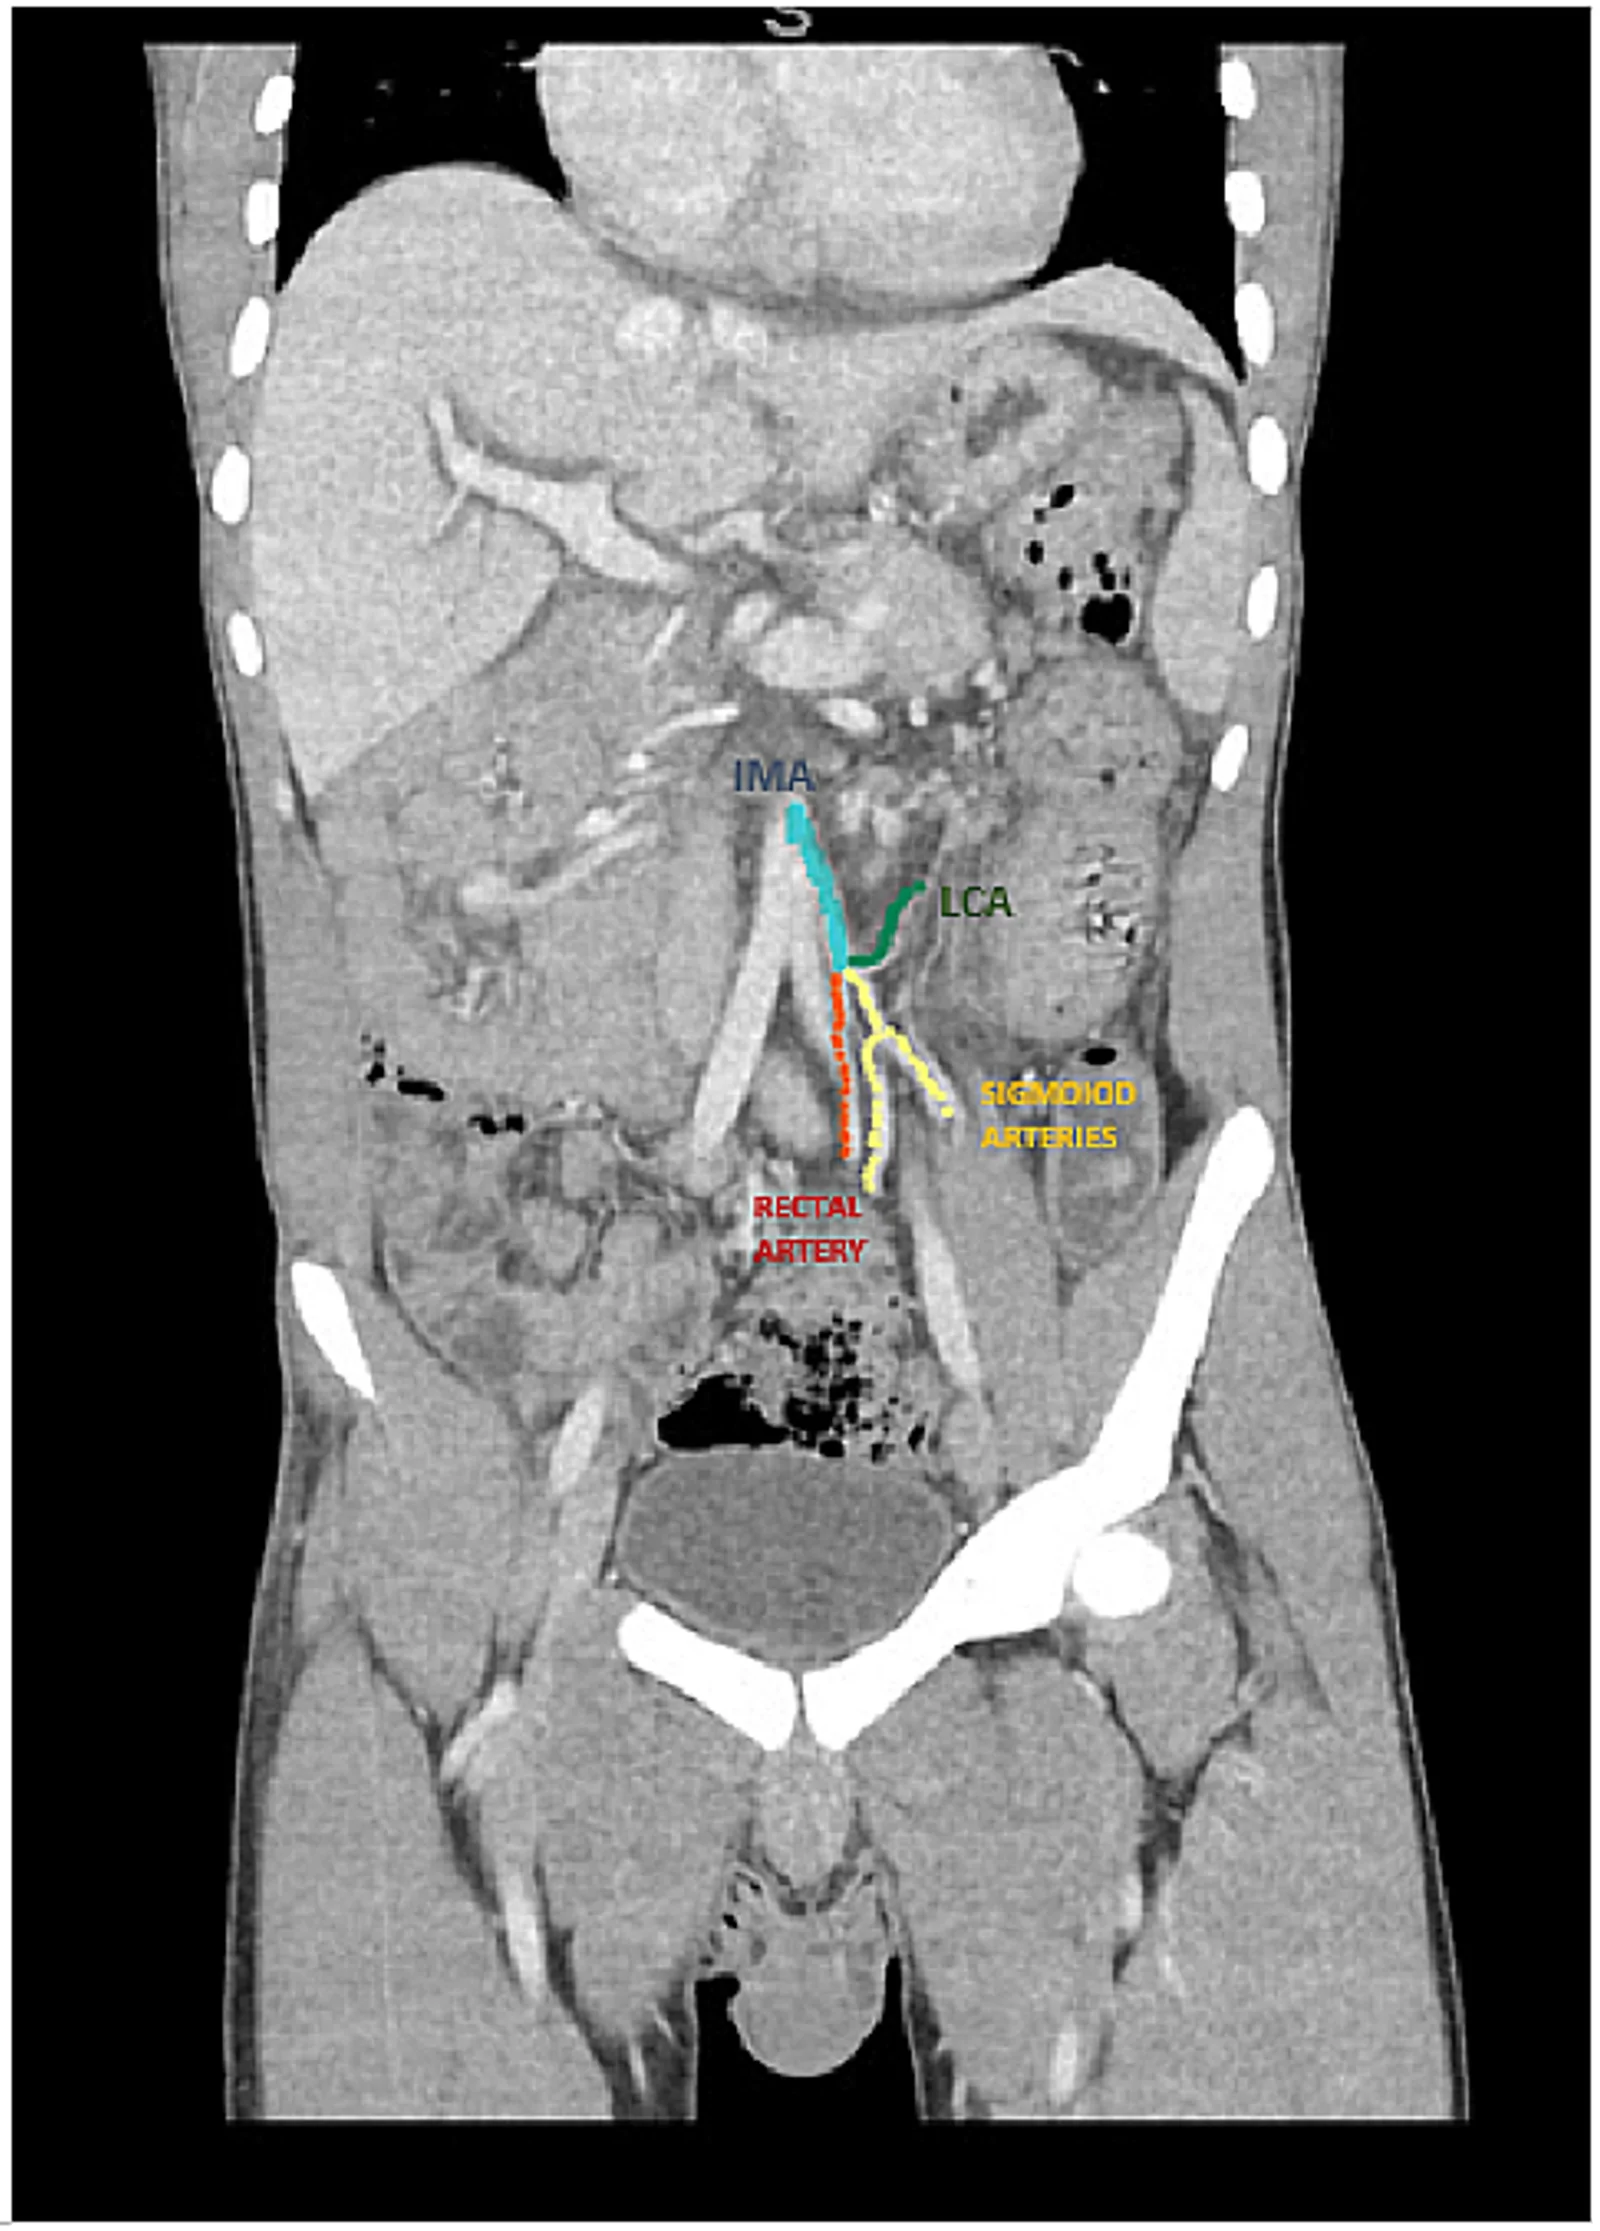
CTA of the abdomen/pelvis showing the IMA, left colic artery, rectal artery, and sigmoid arteries.
